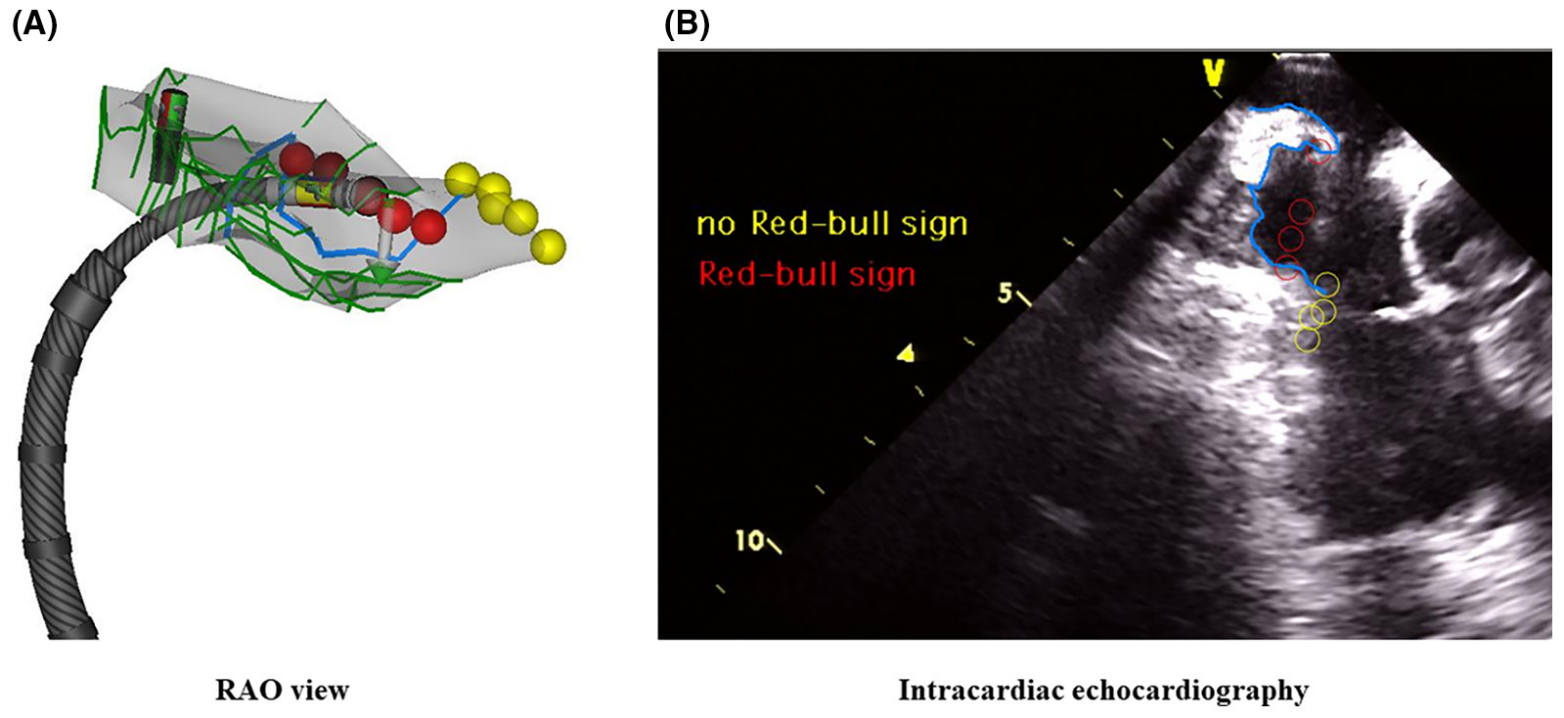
A representative example of the radiofrequency application in the subeustachian pouch (SEP) of cavotricuspid isthmus (A), which is delineated by the intracardiac echocardiography (B). The SEP is delineated as a blue line. A red‐bull sign is observed in all lesions in the SEP. RAO, right anterior oblique.
